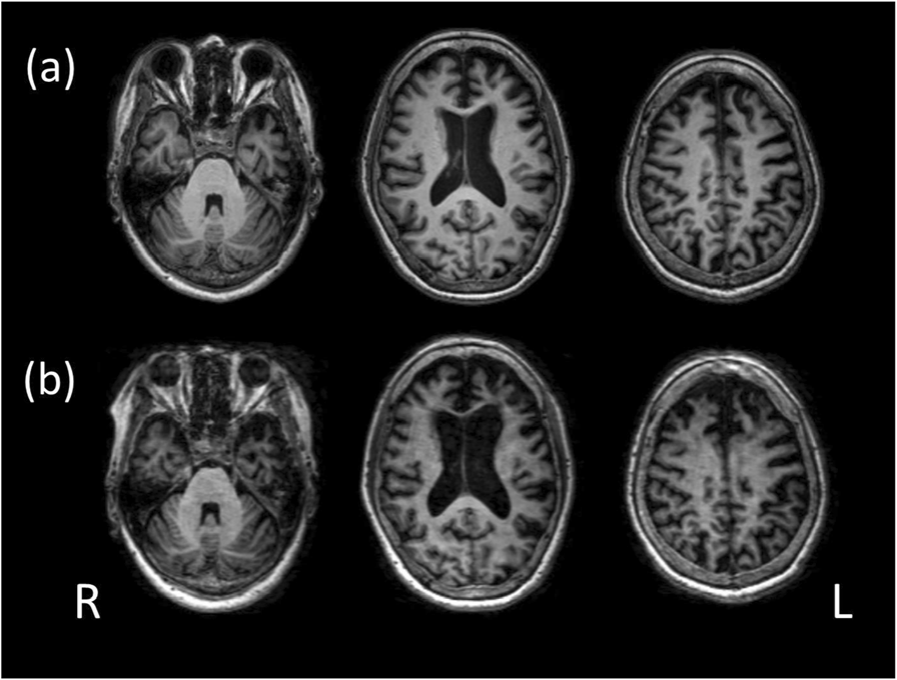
T1-weighed magnetic resonance images showing bilateral frontal and anterior temporal lobe atrophy, as well as bilateral parietal lobe atrophy (a). Atrophy progression 30 months later (b). a has been reprinted with permission from the original publisher (© International Psychogeriatric Association 2017, published by Cambridge University Press) [7]. b has been added to the original images described above. *L* left, *R* right
